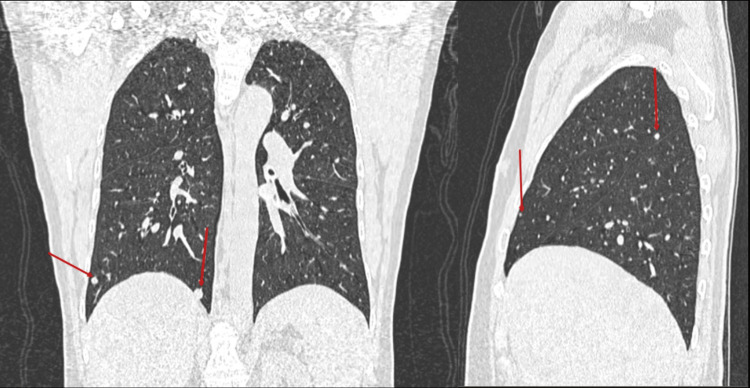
Contrast-enhanced computed tomography (CECT) of the chest in lung window in coronal and sagittal reformats at the level of the aortic arch showing Intraparenchymal pulmonary nodules (red arrows)
